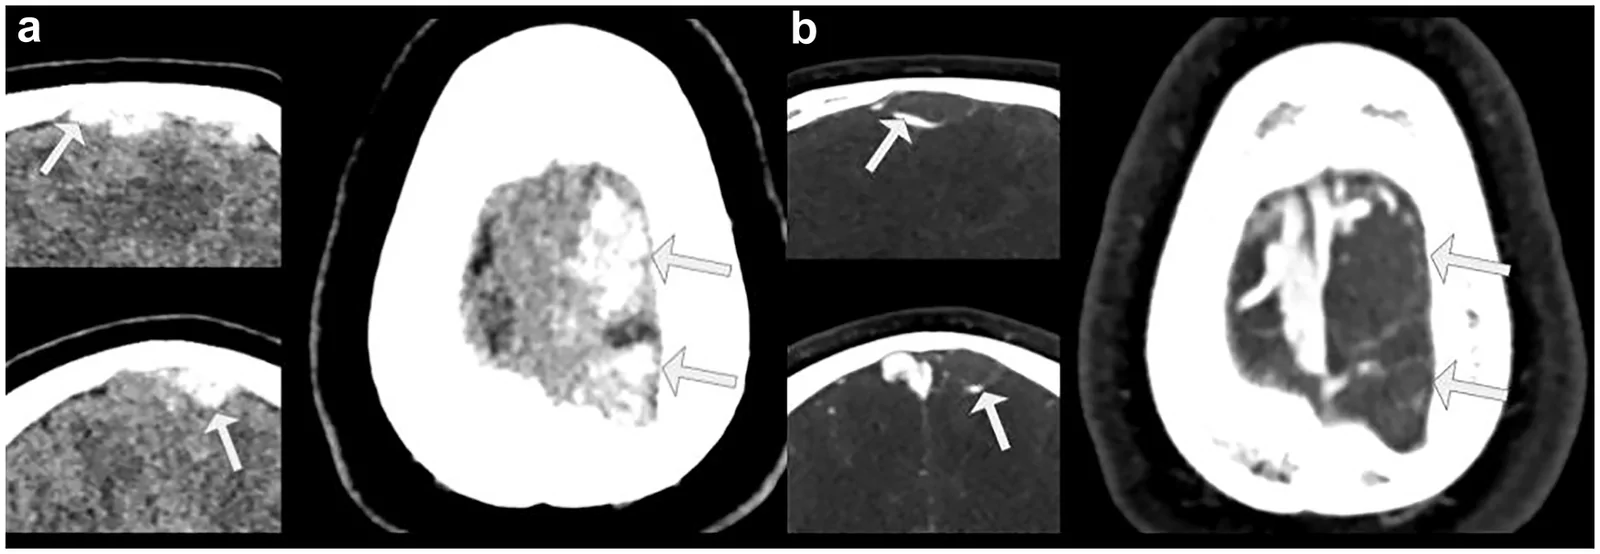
Intracranial venous lake thrombosis (IVLT). a Non-enhanced CT shows hyperattenuating parasagittal structures at the lateral venous lakes (arrows). b CT venography demonstrates absent opacification (arrows), confirming IVLT
